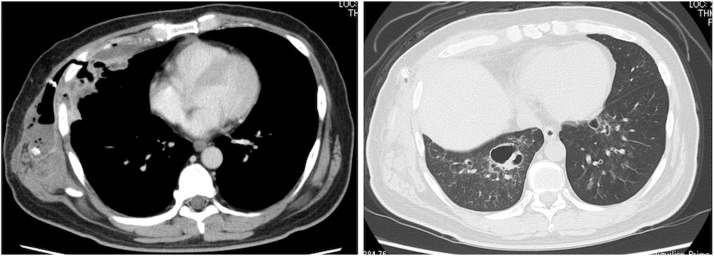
Chest computed tomography on day 11 before open-window thoracotomy.
